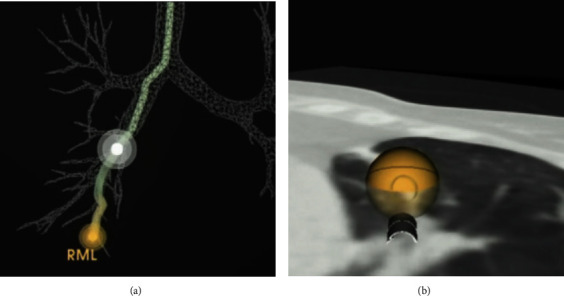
(a) Monarch robotic-assisted navigational bronchoscopy path to the right middle lobe lung nodule. (b) Axial computed tomography imaging superimposed on the robotic-assisted pathway to the right middle lobe lung nodule.
